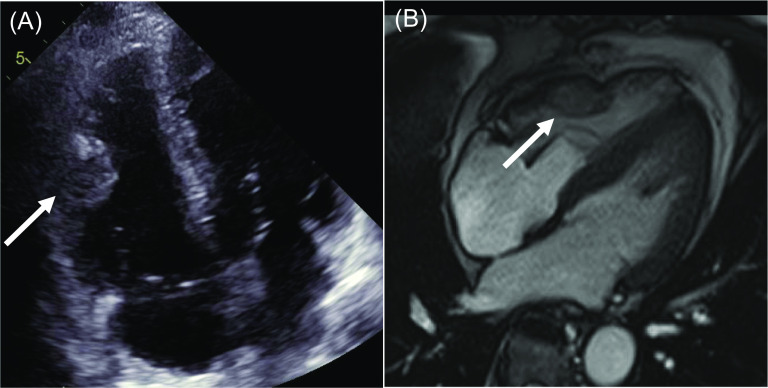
Each white arrow indicates the RV tumor. A Four-chamber view on transthoracic echocardiography. B Cardiac magnetic resonance imaging shows the clear margins of the cardiac tumor. *RV* right ventricular
